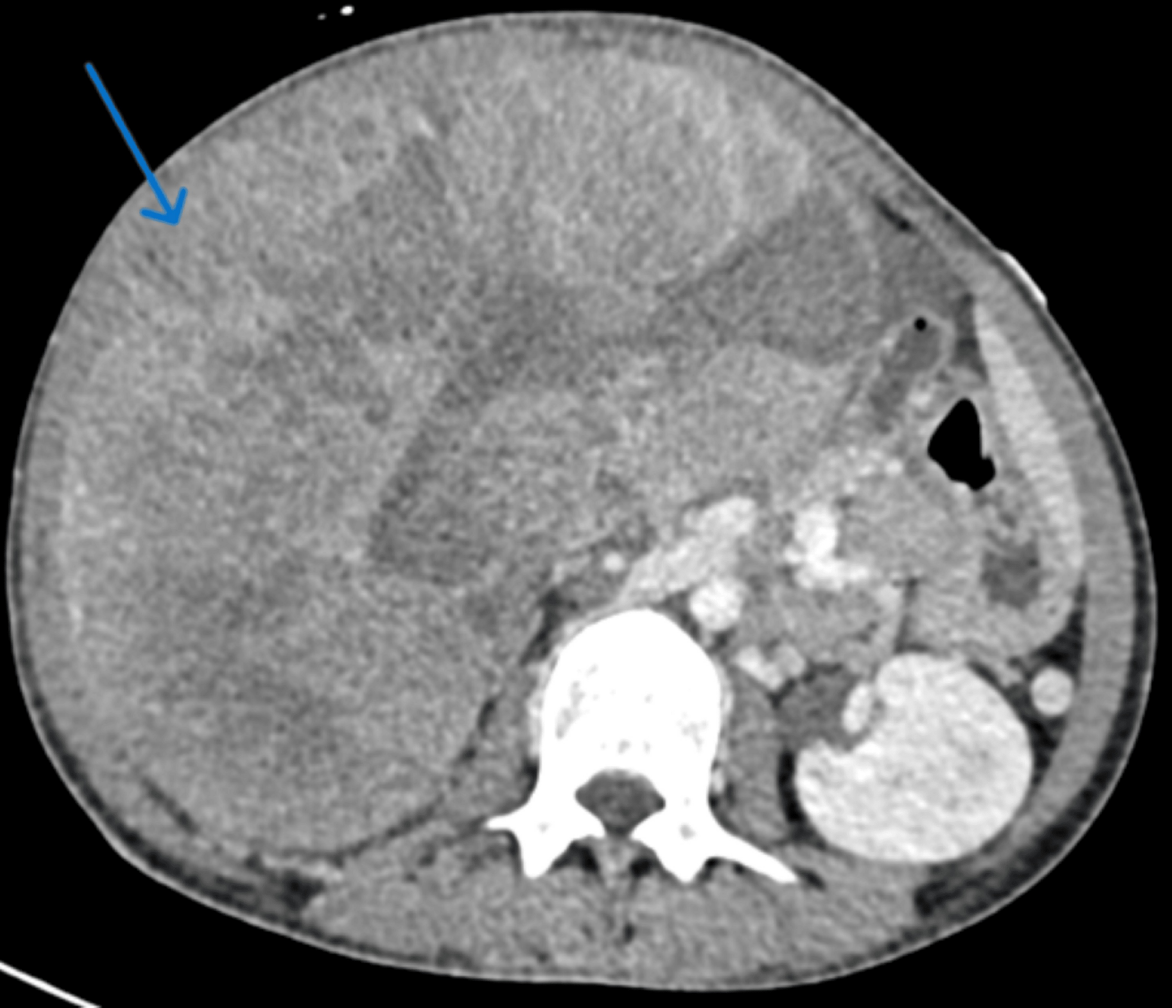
CT scan of the abdomen showing a very large heterogeneous mass
